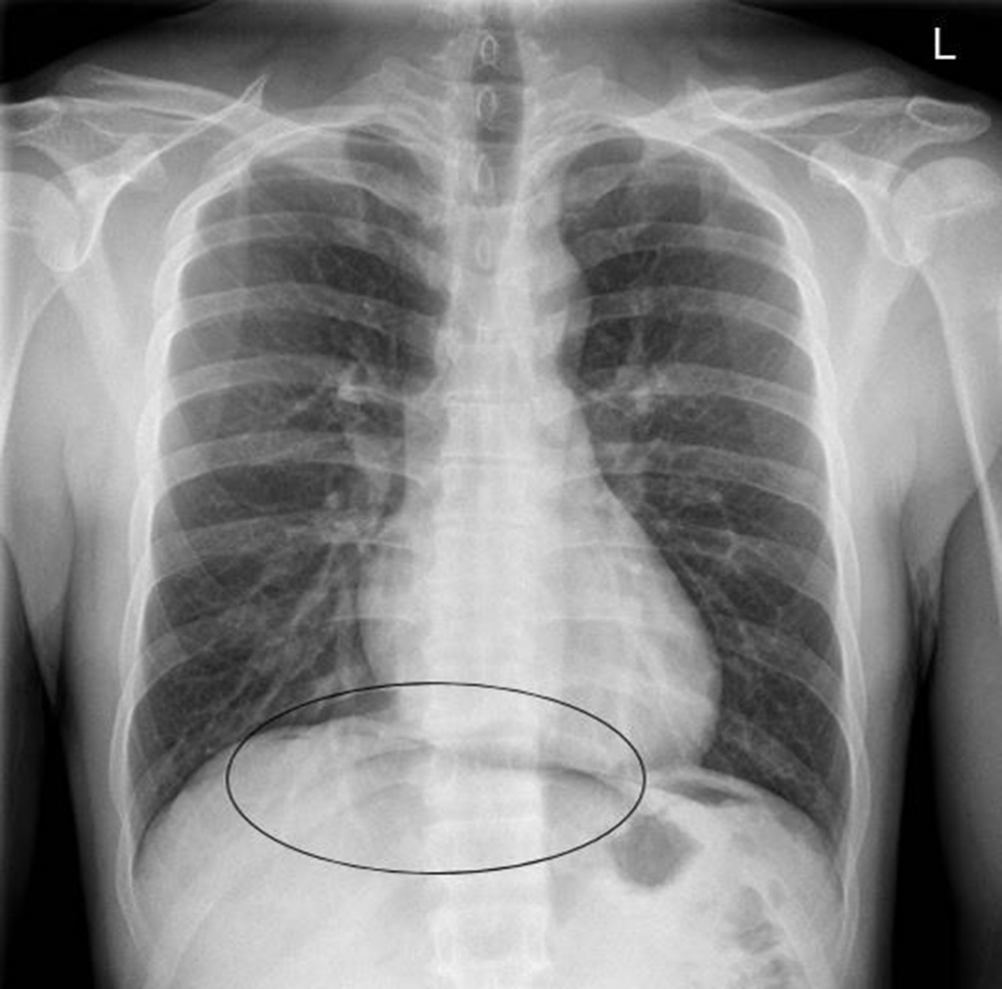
A PA erect chest x-ray; confirming the ultrasound findings and the presence of subdiaphragmatic free air (pneumoperitoneum).
